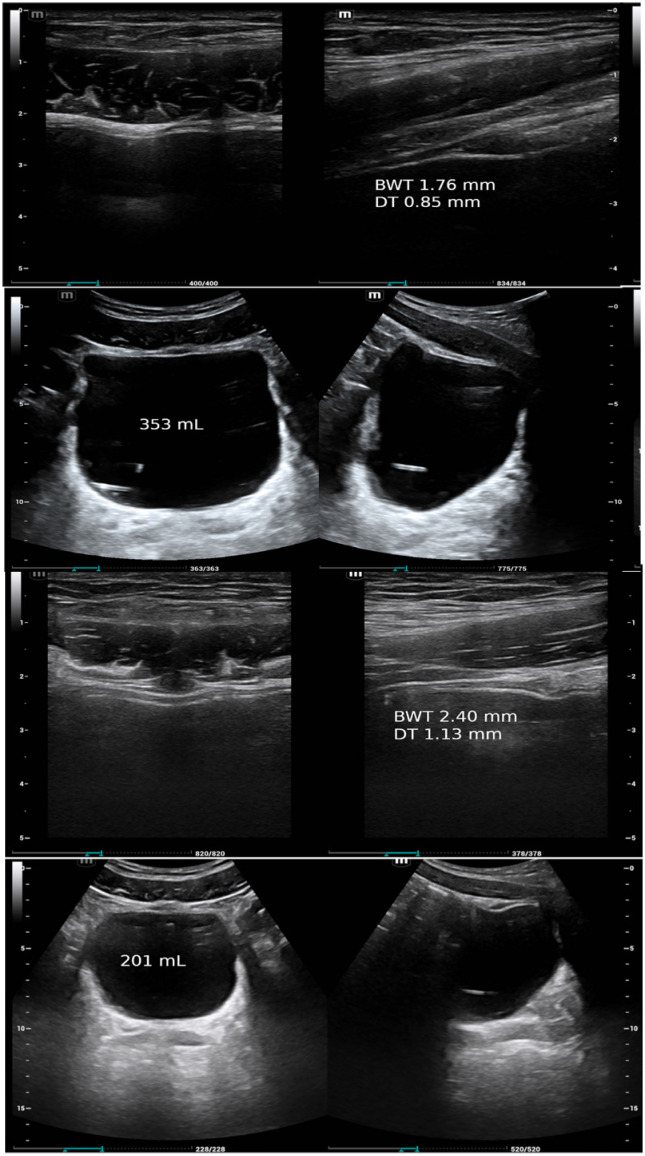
Ultrasonic images showing the layered aspect of the bladder wall in a patient with post-void residual urine (top images). Bladder and detrusor weight were calculated at 42.8 and 18.6 g by our method, compared to 42.3 and 19.3 g by Kojima's. Images obtained during cystometry with a 5 MHz curved array for whole bladder images and with a 10 MHz linear array for the bladder wall. From the first author's archives and used with permission. BWT, bladder wall thickness; DT, detrusor thickness.
